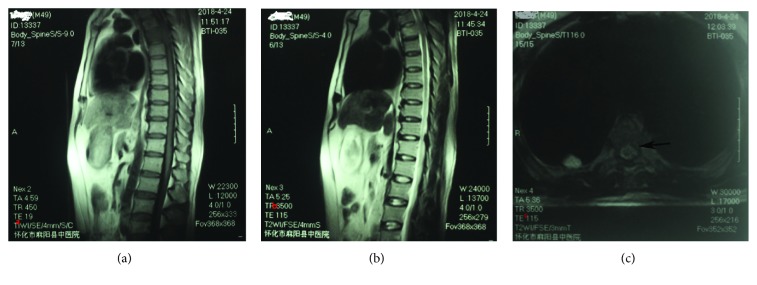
The preoperative thoracic magnetic resonance imaging (MRI) showed a minor intraspinal occupying-lesion and associated pathological fractures of the vertebrae. (a, b) The T1-weighted and T2-weighted images show the fractures of the thoracic vertebrae body. (c) The cross section shows a possibility of an intraspinal space-occupying lesion in the T4 level like the black arrow indicated.
